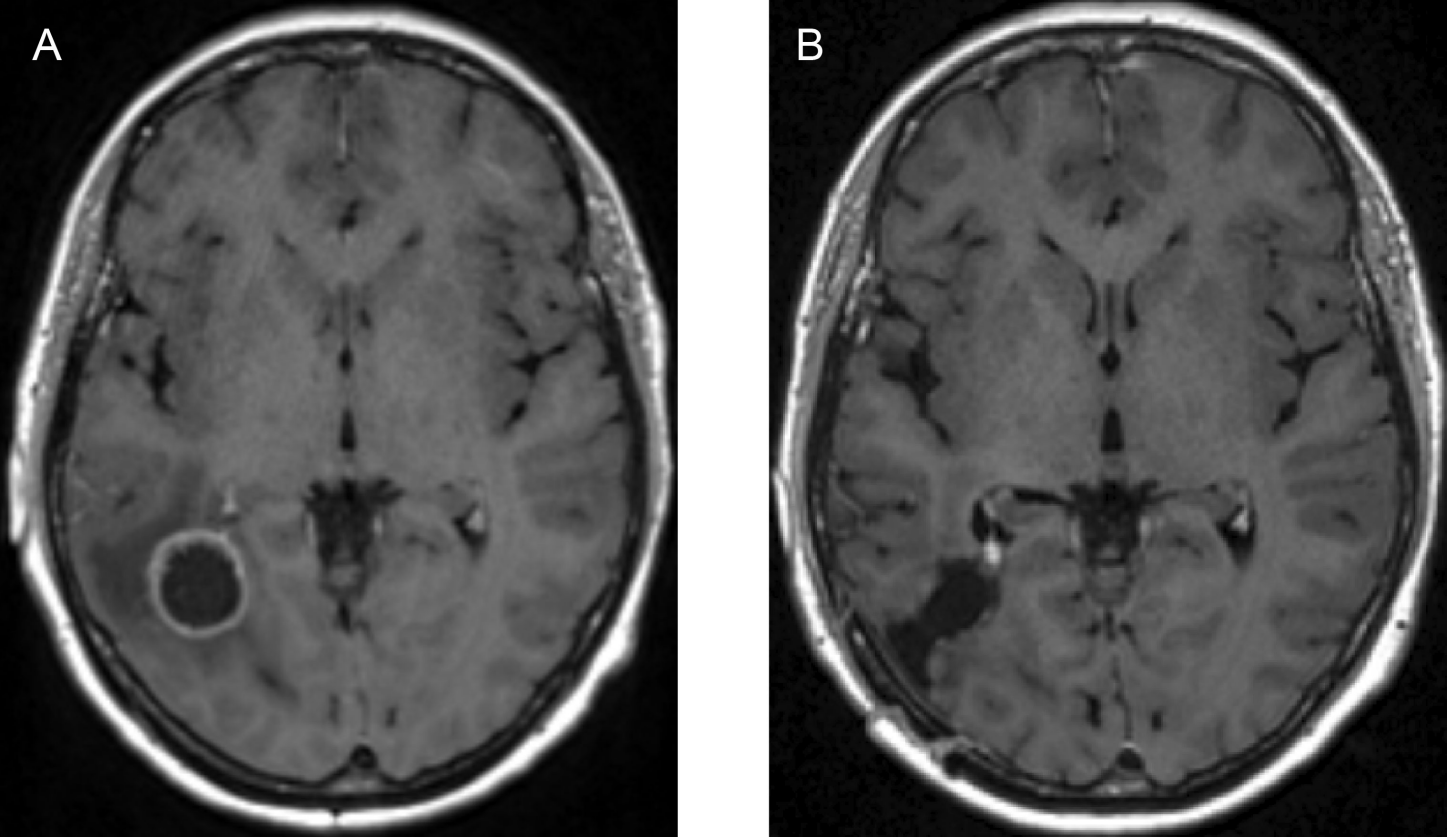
Image showing: (A) axial view of the T1+C pre–operative MRI scan, and (B) axial view of the post–operative MRI scan of the same patient, showcasing the anatomical differences of the brain structure due to the surgical intervention.
